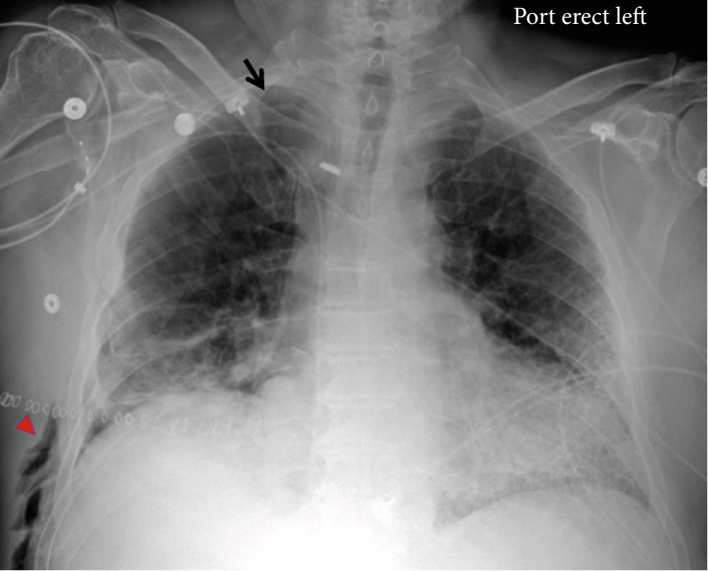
Chest X-ray showed right-sided subcutaneous emphysema (red caret) with a small right-sided pneumothorax (black arrow).
